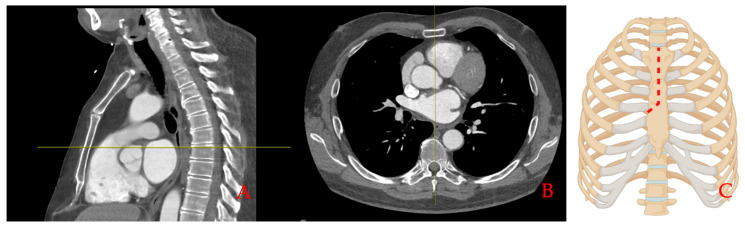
Surgical access in MICS. Surgical planning for MICS: (A,B) envisioned sternum section plane using multiplane CT scan; (C) J-shaped ministernotomy (created with BioRender.com).
